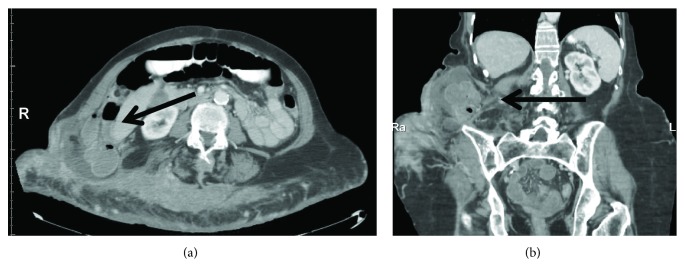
(a, b) CT scan. Bowel herniation through the right posterolateral abdominal wall (arrows).
